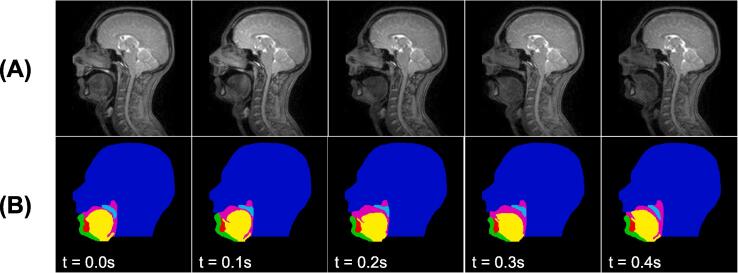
Five consecutive images from one of the series of dynamic two-dimensional magnetic resonance images (A) and ground-truth segmentations of the images (B). The ground-truth segmentations are of the head (dark blue), soft palate (light blue), jaw (green), tongue (yellow), vocal tract (pink) and tooth space (red). t indicates time in seconds. (For interpretation of the references to colour in this figure legend, the reader is referred to the web version of this article.)
